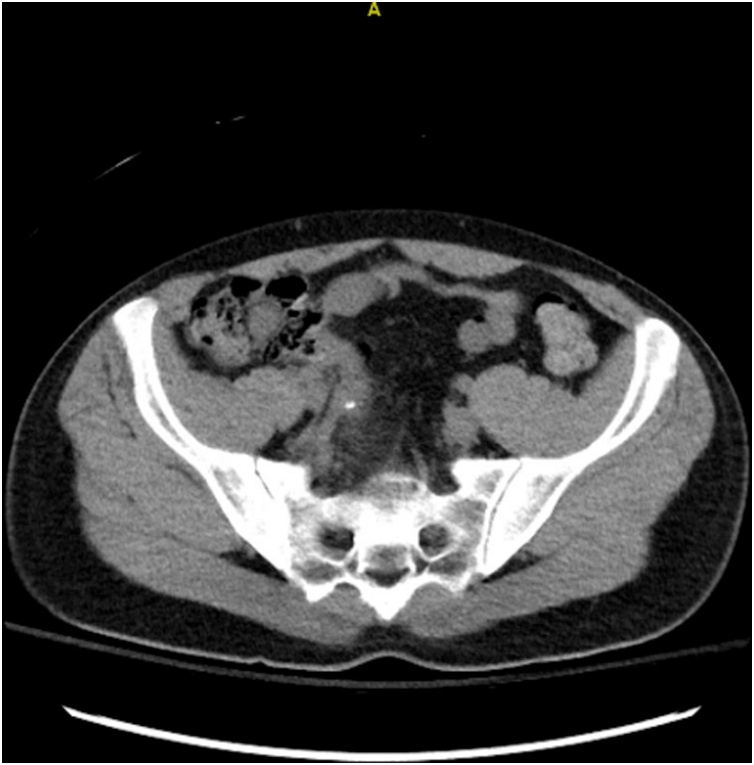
Computed tomography (CT) of abdomen and pelvis showing interval improvement in peri-appendiceal inflammation. Appendicolith is visible at tip of appendix in the right lower quadrant.
